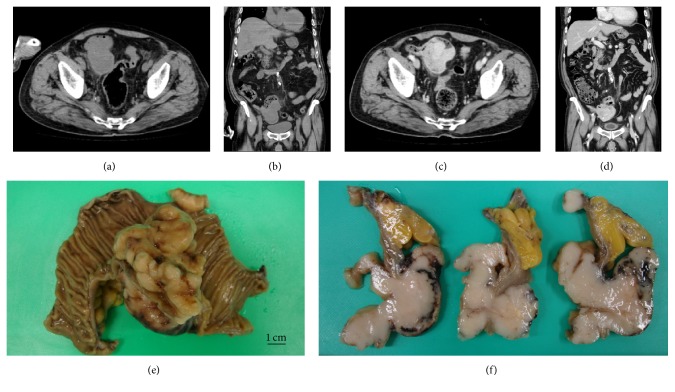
The computed tomography (CT) showed a mass in the small intestine with heterogeneous enhancement. A curative surgery was performed on the 21st day of admission. The tumor had adhered to the retroperitoneum because of localized peritonitis. The tumor size was 60 × 58 × 55 mm. ((a), (b)) Plain CT ((a) axial view, (b) coronal view). ((c), (d)) Enhanced CT ((c) axial view, (d) coronal view). (e) Gross appearance of the resected mass. (f) sections of the mass. Scale bar = 1 cm.
